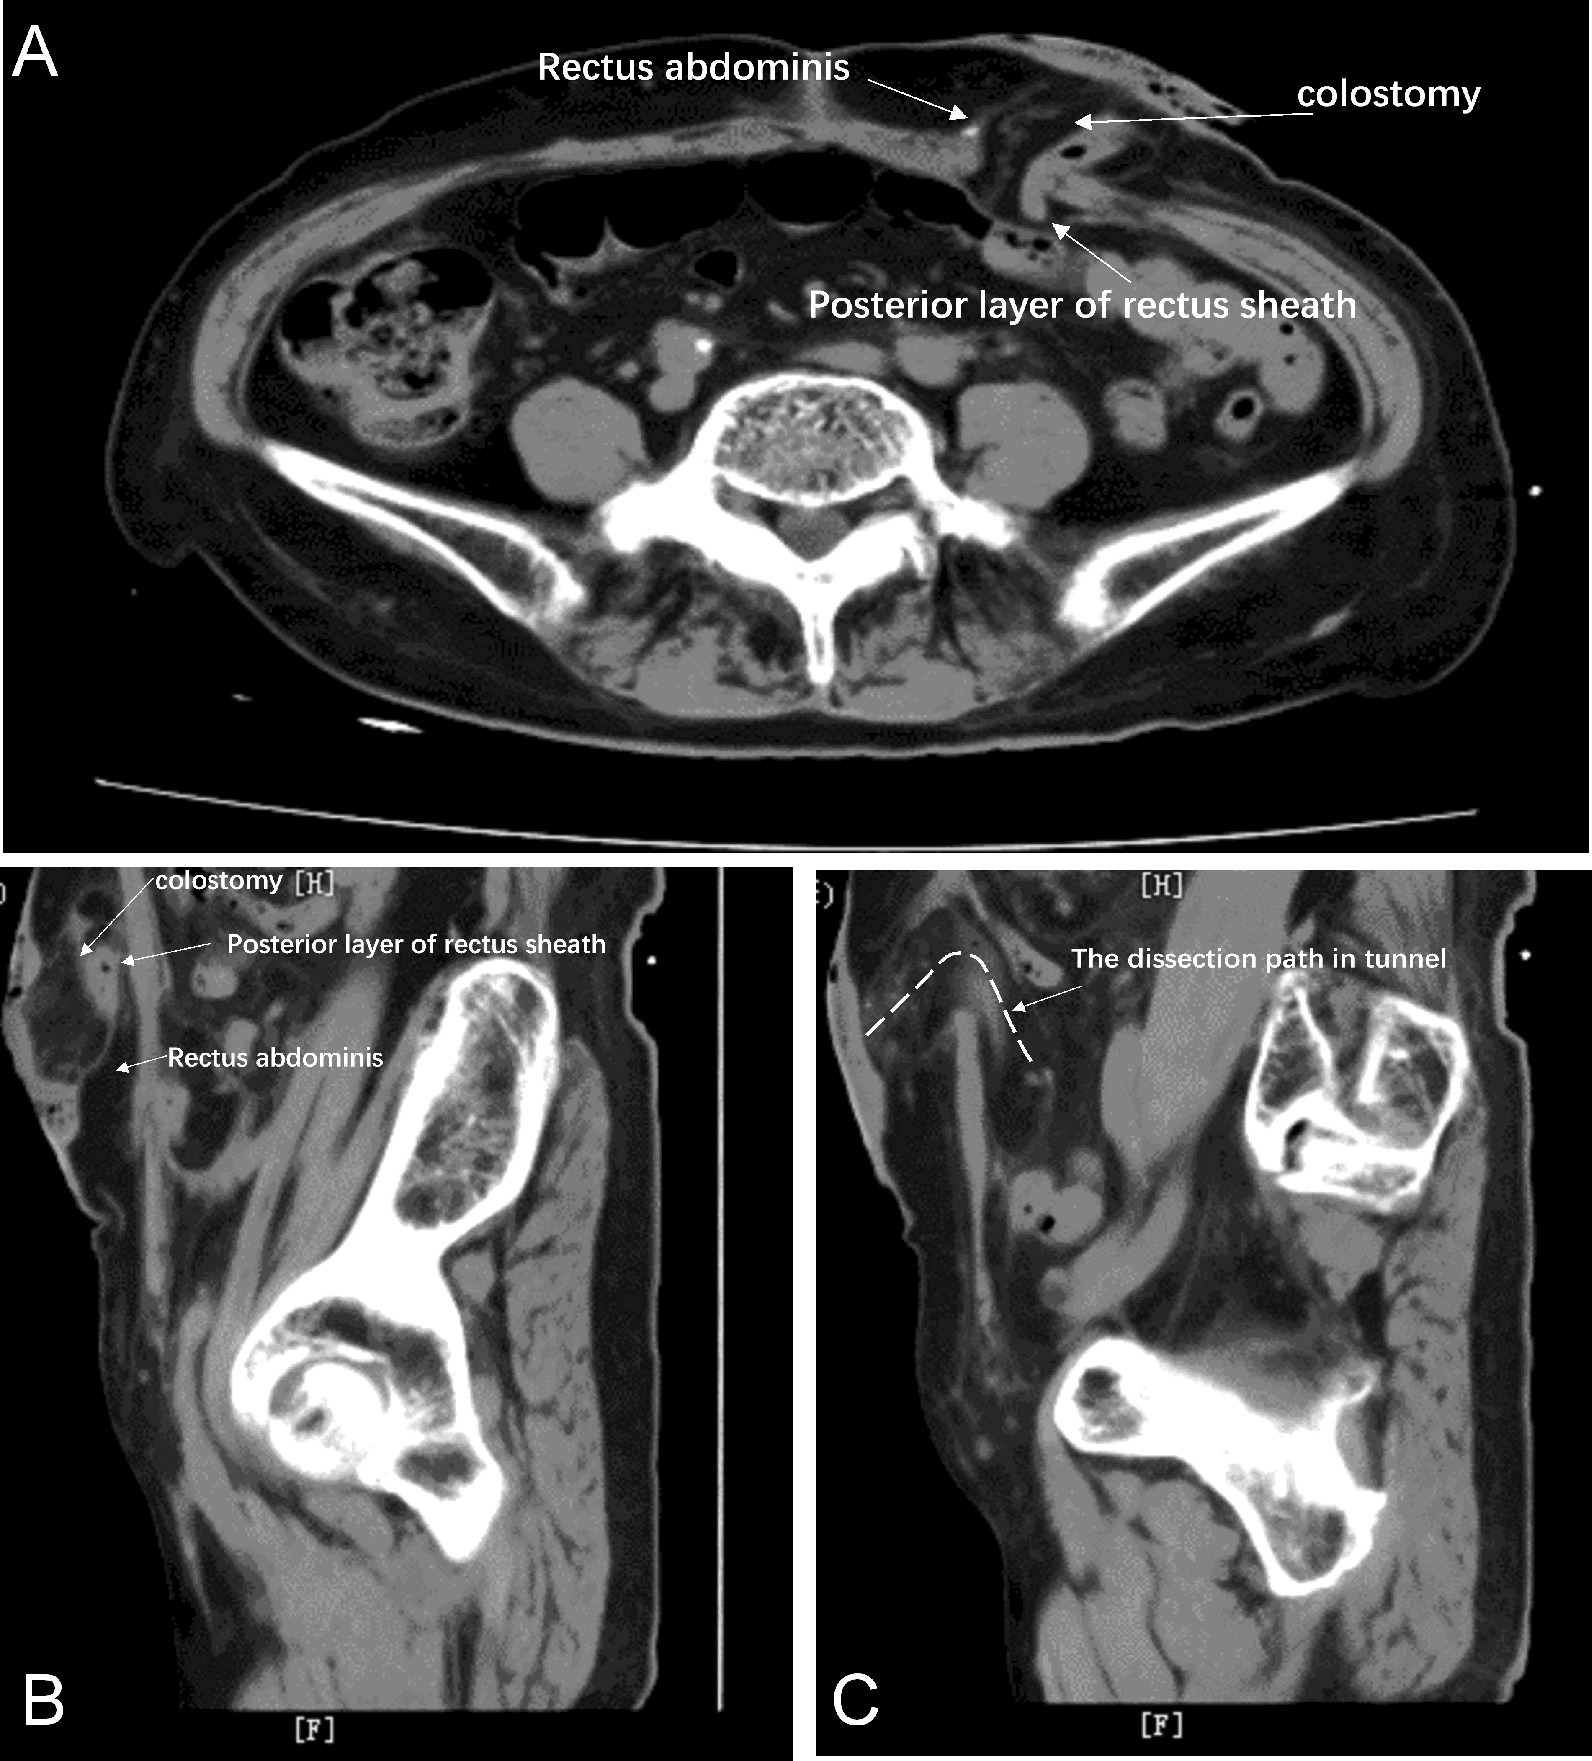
CT scan at 1 month after APR
